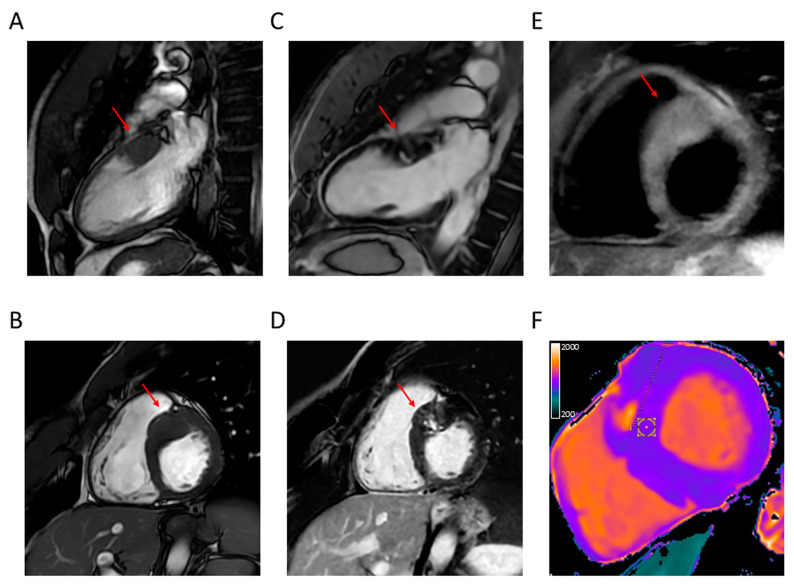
Representative CMR images from a male patient aged 26 years with typical asymmetric basal septal hypertrophy associated with fibrosis. (A) two-chamber long axis and (B) short axis view showing asymmetric basal septal hypertrophy (arrow); (C) two-chamber long axis and (D) short axis view showing LGE with patchy distribution at the level of the hypertrophic segment (arrow); (E) short axis T2-weighted view showing hyperintensity at the same level (arrow); and (F) short axis view showing increased T1 mapping at the same level. CMR = cardiac magnetic resonance; LGE = late gadolinium enhancement.
